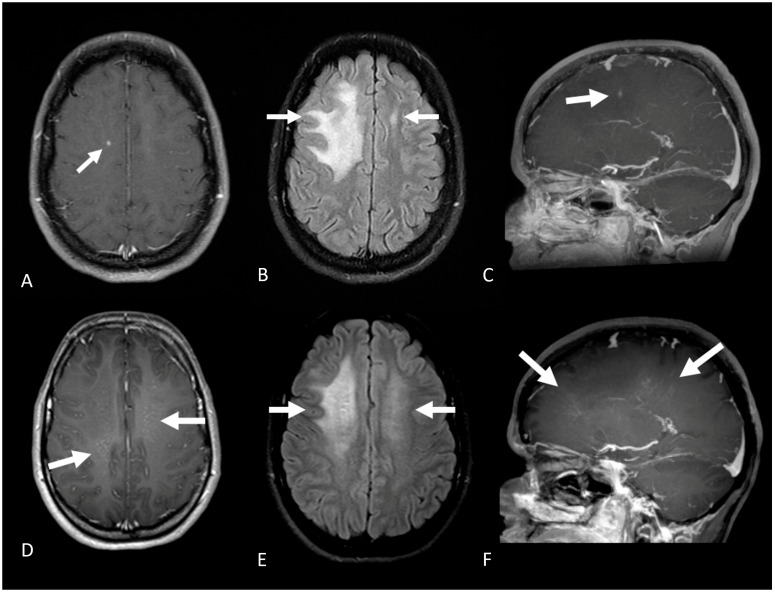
Axial (A) T1 post-contrast brain MRI, (B) axial FLAIR and (C) sagittal post-contrast T1 weighted MRI show a punctate enhancing lesion in the right superior frontal gyrus with adjacent vasogenic edema (white arrows). After treatment, the small punctate lesion disappeared (D) with reduction of the vasogenic edema (E); however, multiple linear enhancing lesions with perivascular distribution were seen in the deep white matter (white arrows in (F)).
